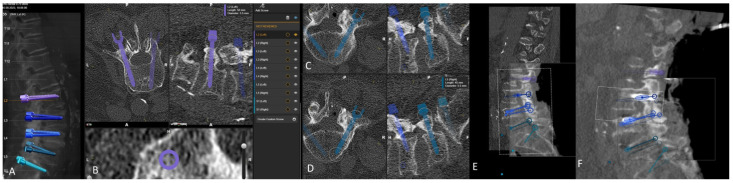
Preoperative planning of PS for stabilization of L2-S1 according to preoperative CT in (A) sagittal view after automatic detection of vertebrae and (B) planning of the position, length, and diameter of each screw in axial, sagittal, and perpendicular view. (C) Axial and sagittal view of the right L5 screw with automatic planning—note that the screw tip is aligned towards the endplate. (D) Position of L5 screw right after manual repositioning. (E) Fusion of preoperative CT and iCT registration scan. (F) Fusion of the registration and control iCT scan.
